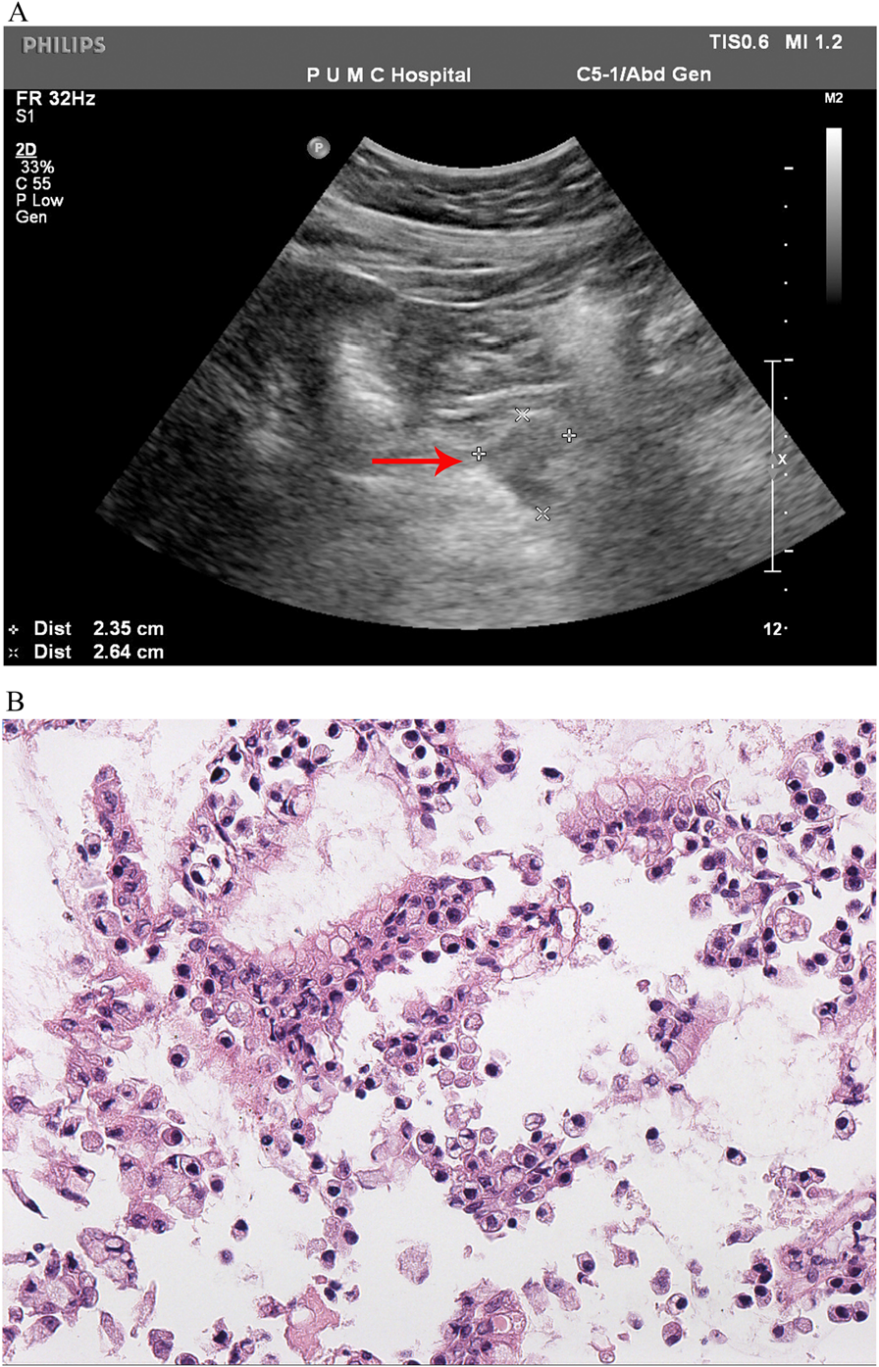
A Abdominal ultrasound showed a hypoechoic mass (red arrow) which tended to protrude outward at the head of the pancreas. (B) Cell block of fine needle aspiration showed mucinous columnar cells with mild dysplasia and scattered plasmacytoid cells with salt-and-pepper chromatin (HEx200)
